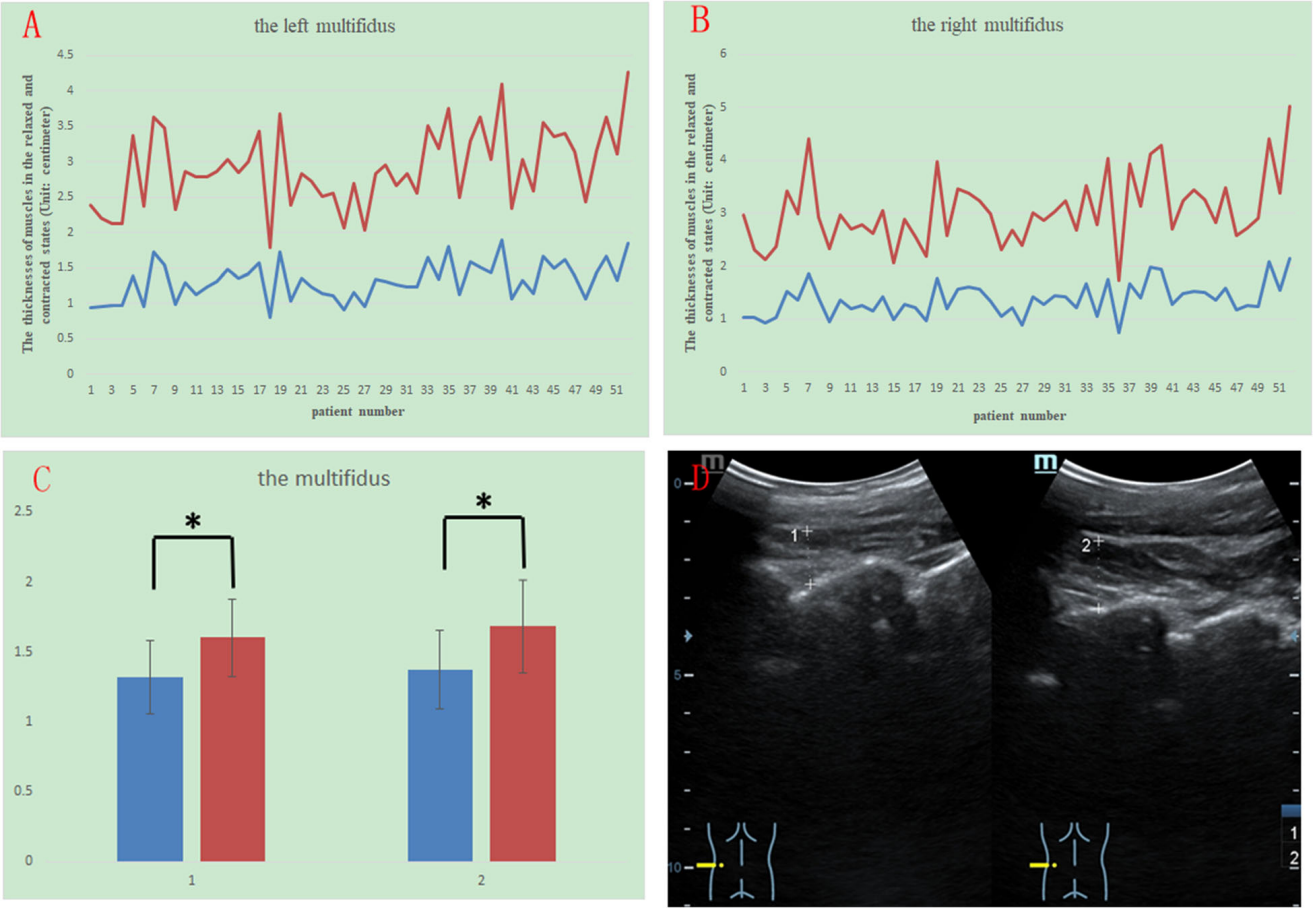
The thicknesses of the left (Fig. 2 A) and right (Fig. 2B) multifidus in the resting (blue line) and contracted conditions (red line). Comparison of the thicknesses in the resting (blue column) and contracted conditions (red column). Group 1 is right, and group 2 is left (Fig. 2 C). The sonogram of the multifidus: left shows the relaxed state, and right shows the contracted state (Fig. 2D)
